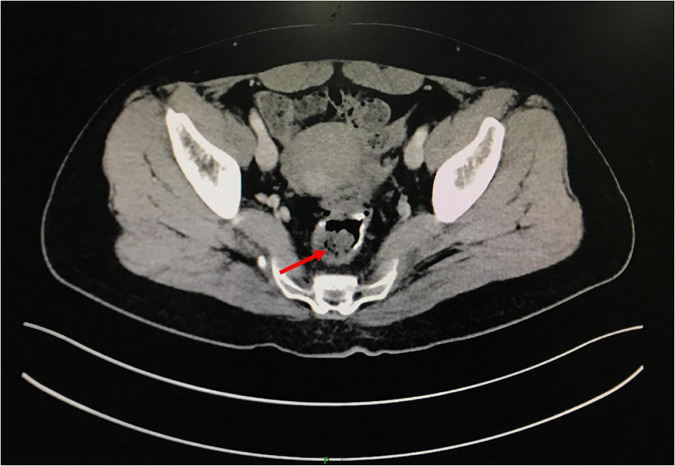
The pelvic cavity CT image.
